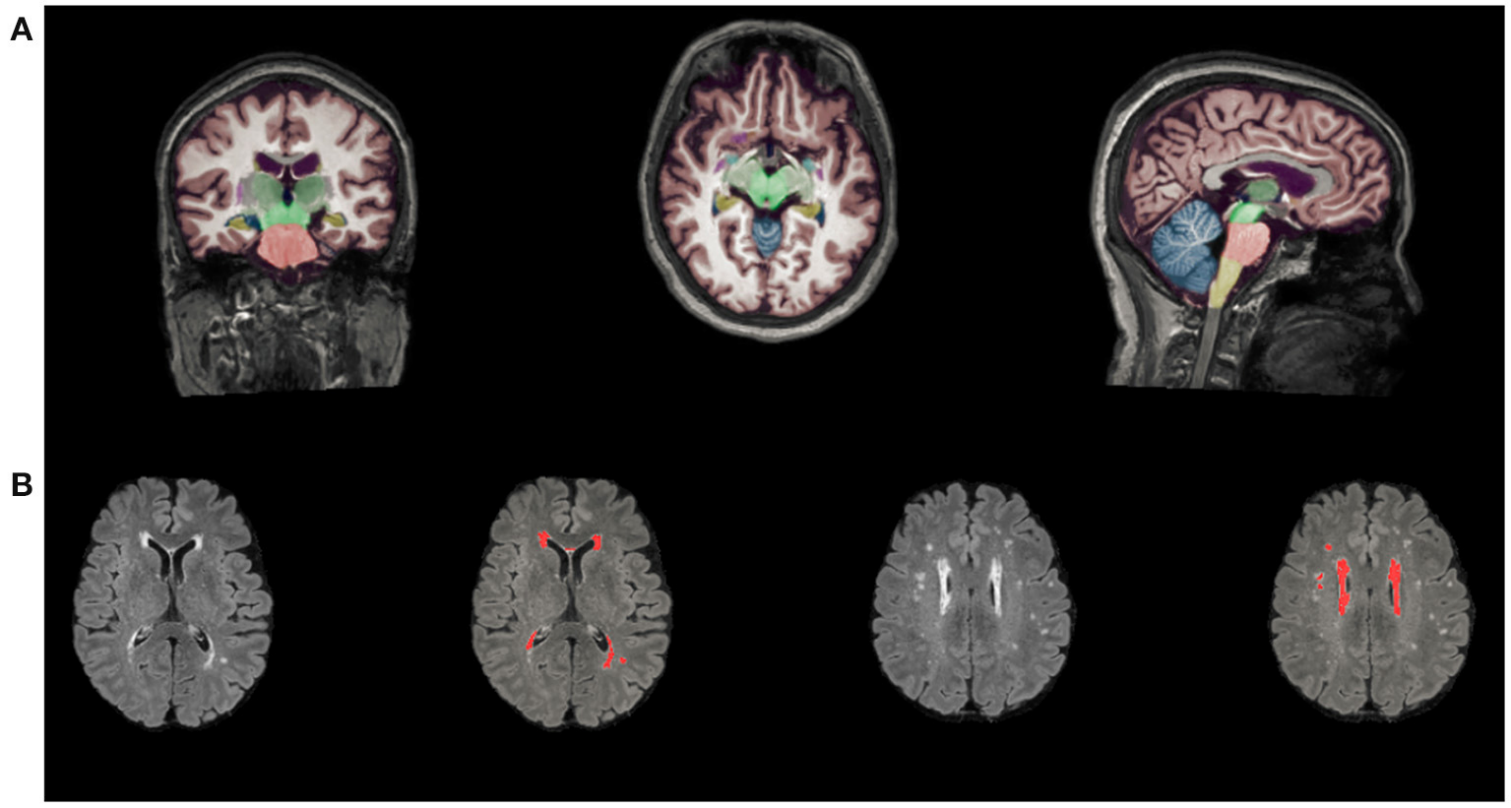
Automated segmentation and quantitative using AccuBrain™. (A) Volume segmentation and quantification of anatomical regions based on T1-weighted MRI scans. (B) WMH volume segmentation and quantification were based on FLAIR images. WMH, white matter hyperintensities.
